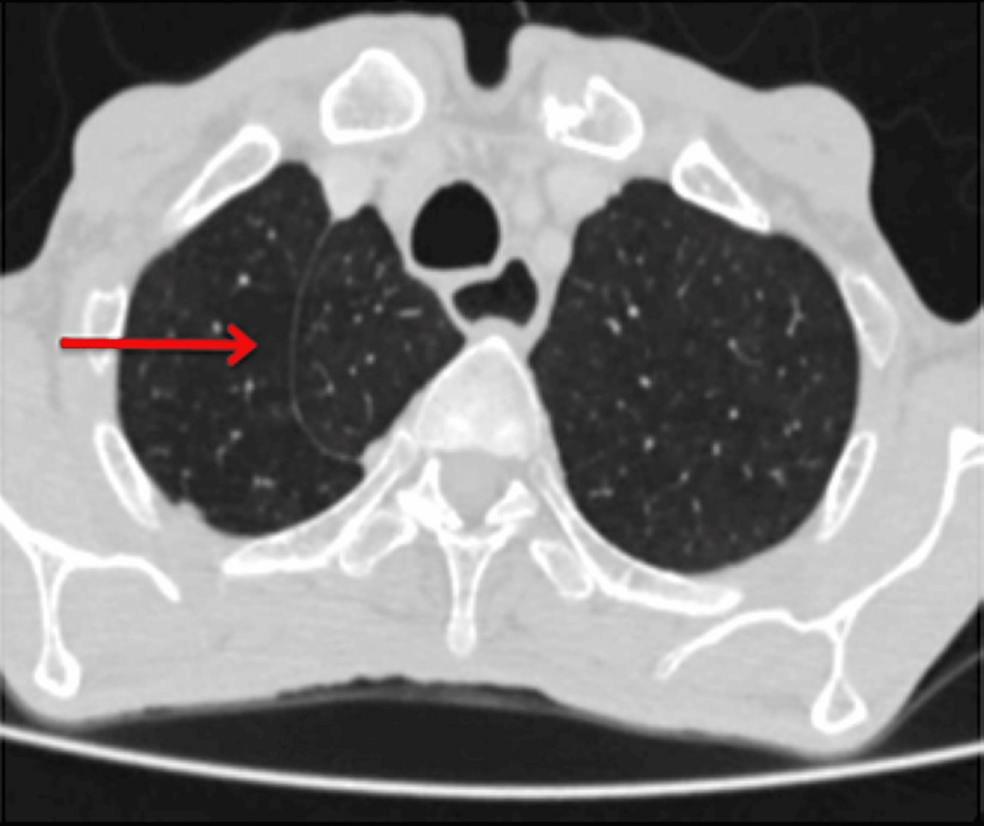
High-resolution computed tomography (HRCT) chest axial image shows curvy-linear white line (red arrow) in the right upper lobe, the azygos fissure.
